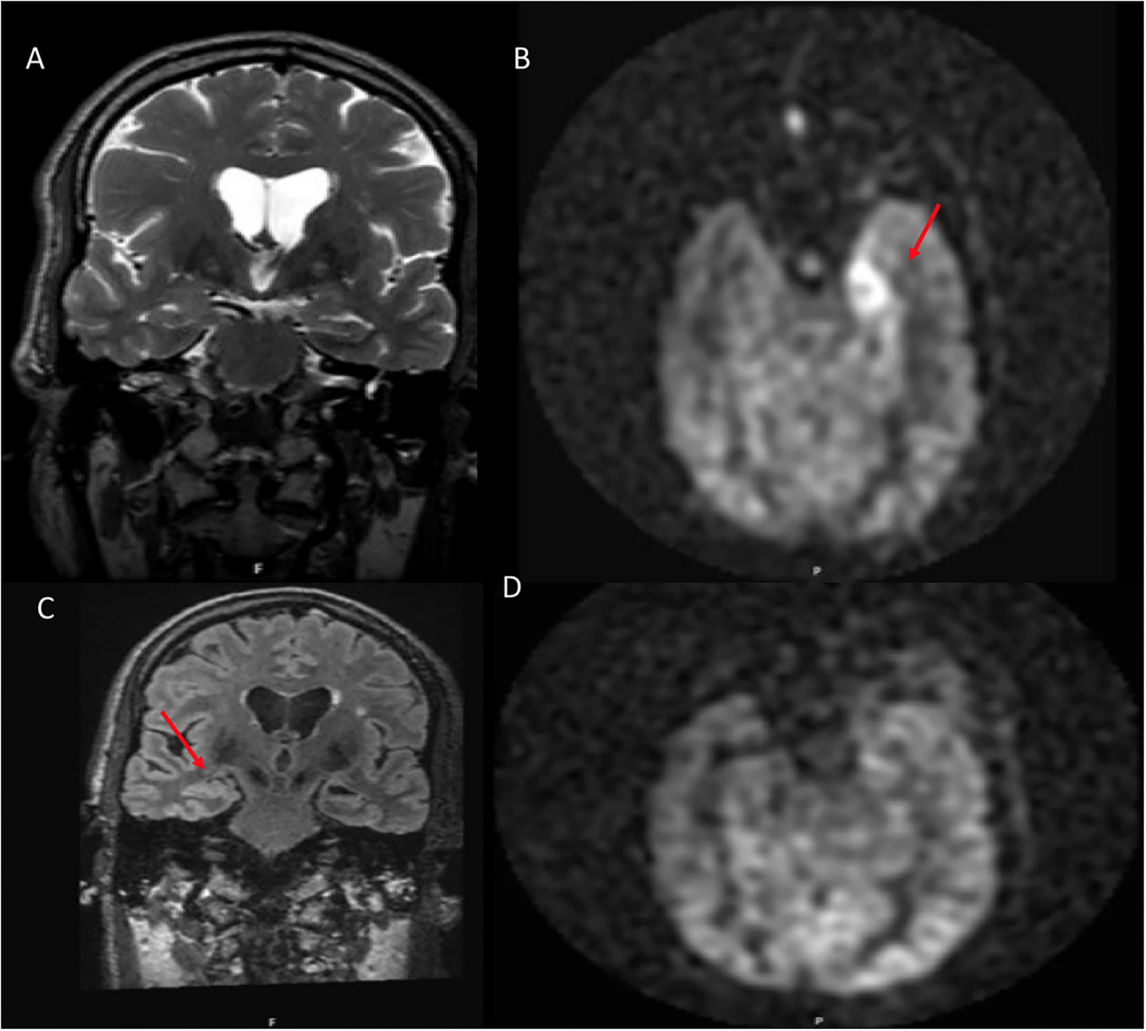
A 54 year old male with traumatic brain injury. (A) Coronal T2W shows no structural abnormality. (B) ASL demonstrates hyperperfusion within the left amygdala. (C) 8 month follow up coronal FLAIR shows left hippocampal volume loss. (D) ASL demonstrates resolution of the previous hyperperfusion.
